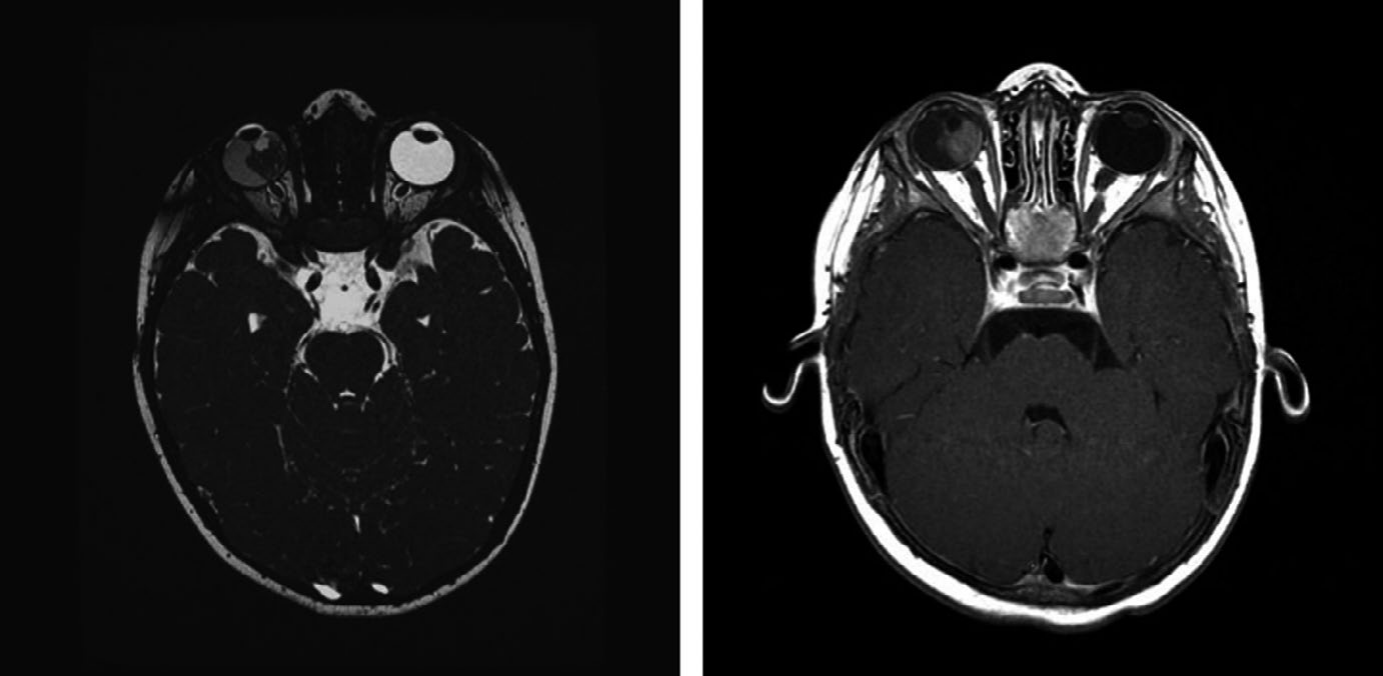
Baseline brain MRI at age 15 months
